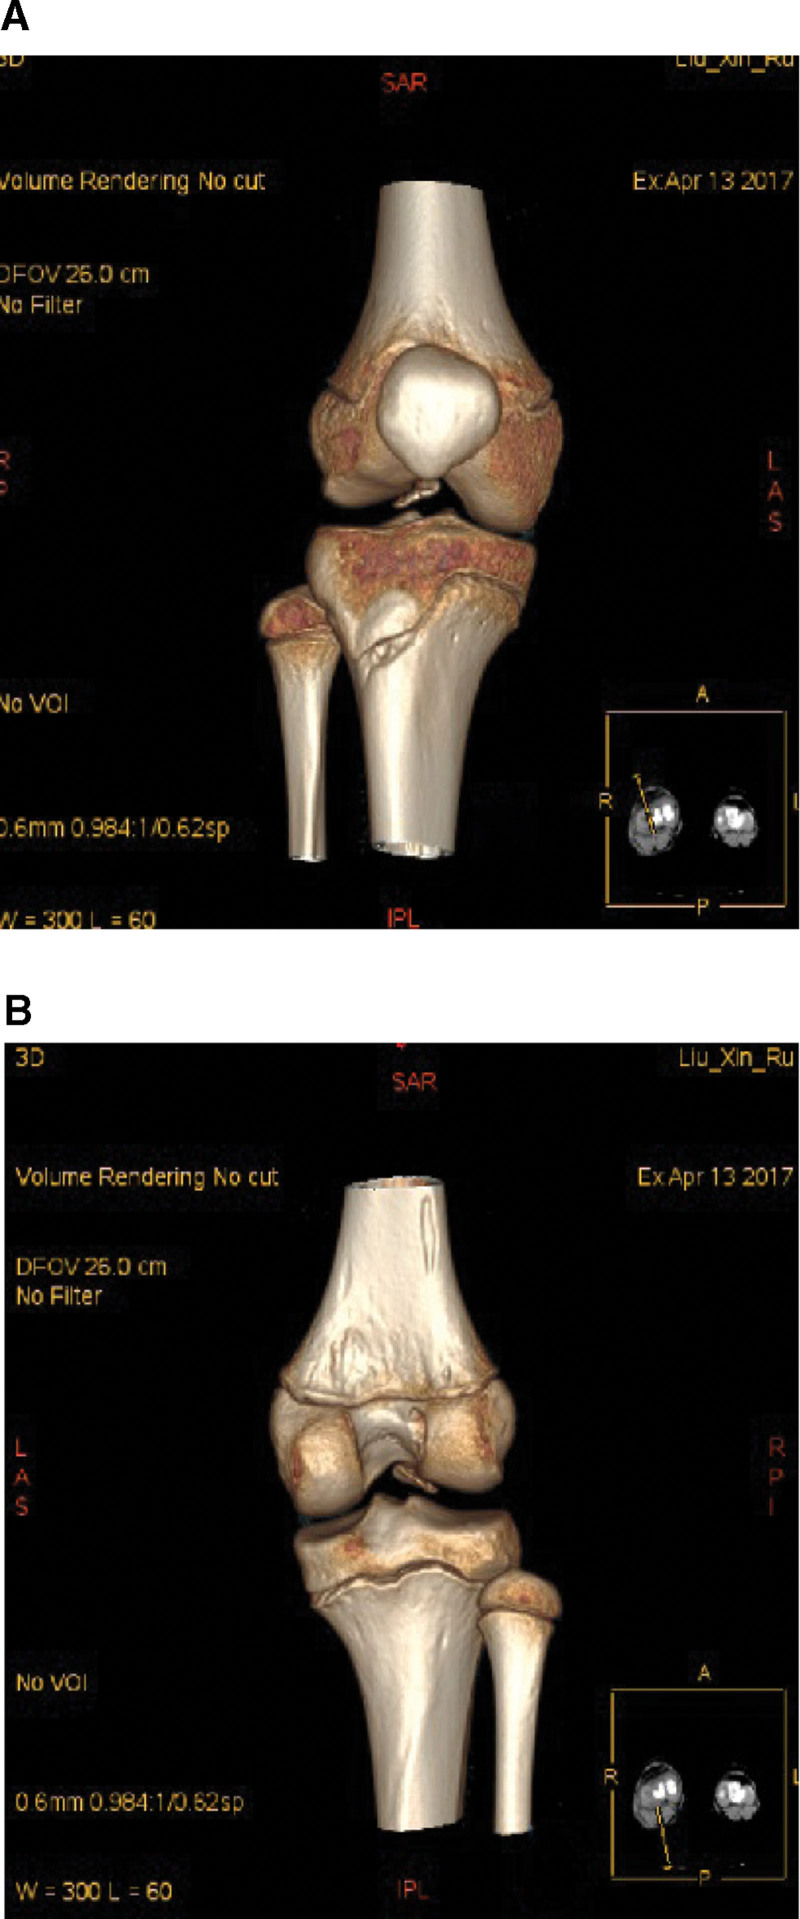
(A, B) CT scan image clearly shows that the lateral femoral condyle had been fractured posteromedially within the intercondylar notch at the proximal ACL junction.
